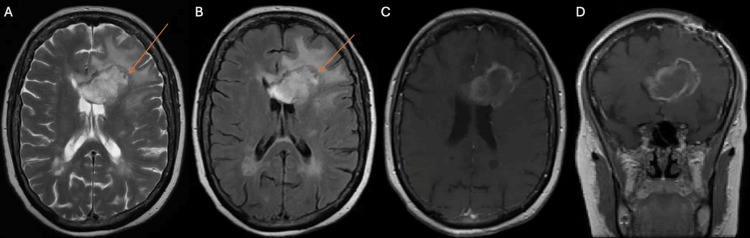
Post-biopsy MRI. Axial T2-weighted MRI shows a hyperintense signal surrounding the bilateral frontal horns, accompanied by evidence of partial subfalcine herniation (A). Axial fluid-attenuated inversion recovery imaging reveals subependymal edema in the ventricular system, with hyperintensity localized to the left centrum semiovale (B). Axial T1-weighted MRI with gadolinium enhancement reveals an irregular lesion in the left frontal lobe (C), resulting in the collapse of the frontal horns on the coronal view (D). In images A and B, a notch from the biopsy can be observed at the periphery of the lesion (orange arrow).
